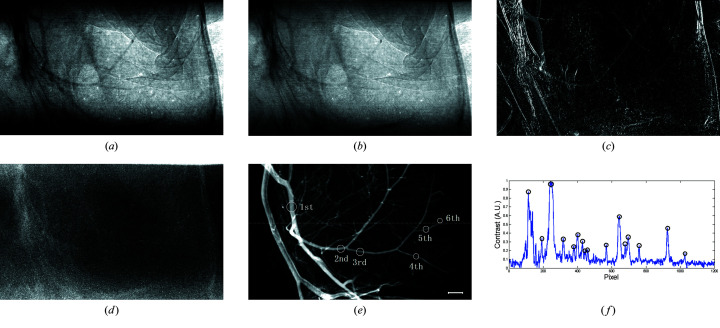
The experimental results of MCXI with synchrotron X-rays, where (*a*) represents the single image frames of the raw angiogram, (*b*) is the time-averaged image of the raw angiogram, (*c*) is the image of moving tissues reconstructed by MCXI, (*d*) represents the high-frequency noises reconstructed by MCXI, (*e*) represents the intact blood vessels delineated by MCXI and (*f*) is the intensity profile along the horizontal dashed line in Fig. 3 ▸(*e*). The scale bar represents 500 µm.
